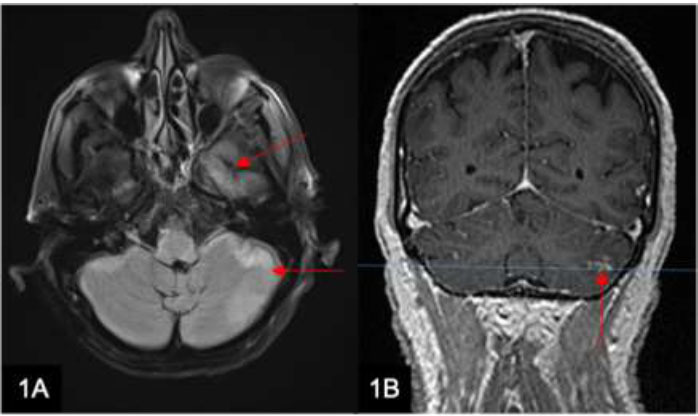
A 63-year-old man with a history of esophageal cancer presents with new-onset flaccid paraparesis and fever. Axial T2-weighted (w) FLAIR (A) and Coronal T1w post-contrast (B) MRI brain images show gyriform T2 hyperintensities along the left temporal lobe and left cerebellar hemisphere with leptomeningeal enhancement (arrows). These features are consistent with metastatic leptomeningeal carcinomatosis.
